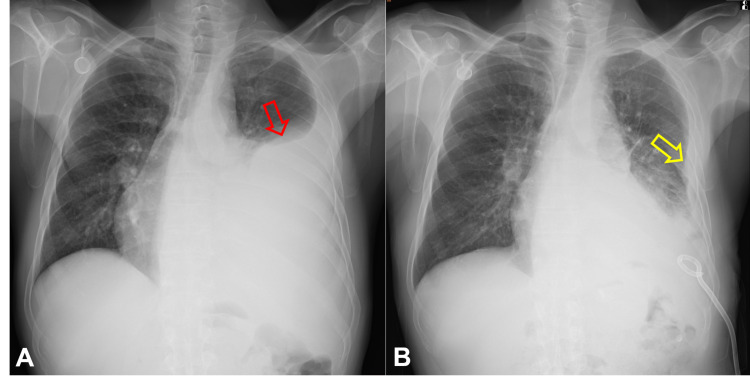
Chest X-ray films of the patient at presentation. A. Left side massive pleural effusion (red arrow) (May 6, 2020). B. Pleural thickening clearly seen (yellow arrow) after pleural effusion drainage with a pigtail catheter (May 18, 2020).
